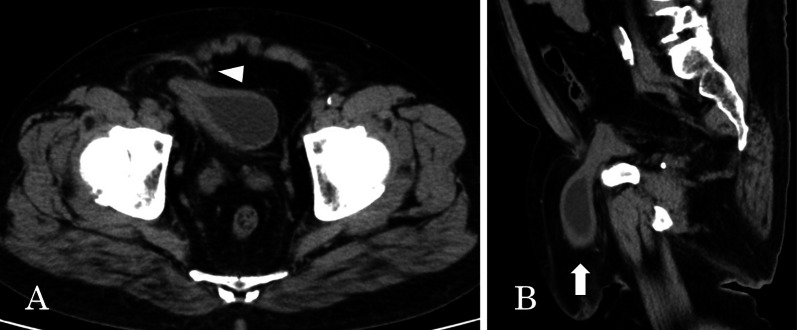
CT scan findings. Abdominal and pelvic CT scans show the right indirect inguinal bladder hernia. A A part of the bladder protruded outside the inferior epigastric vessels (white arrowhead). B The protruded bladder (white arrow) containing urine ran into the scrotum
